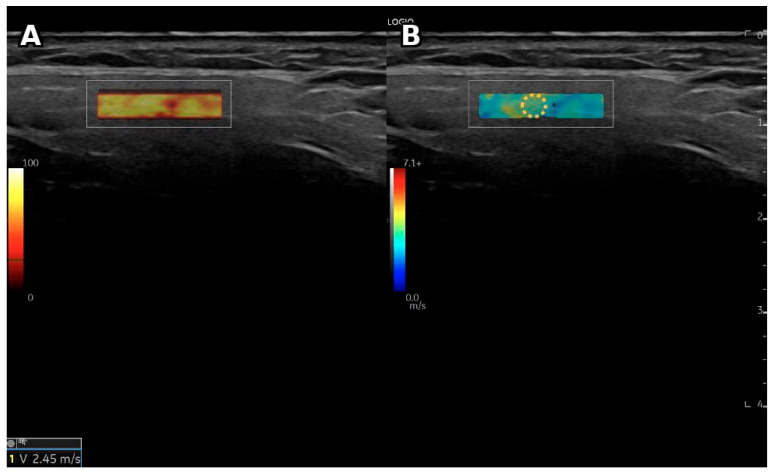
Representative B-mode ultrasonography and shear-wave elastography (SWE) images of the parotid gland in a patient with primary Sjögren’s syndrome. (A) B-mode ultrasonography showing heterogeneous parenchymal echotexture of the parotid gland. (B) Corresponding SWE velocity map demonstrating increased tissue stiffness within the region of interest (ROI). The dotted circle indicates the region of interest (ROI). Warmer colors indicate higher shear-wave velocity values (m/s).
